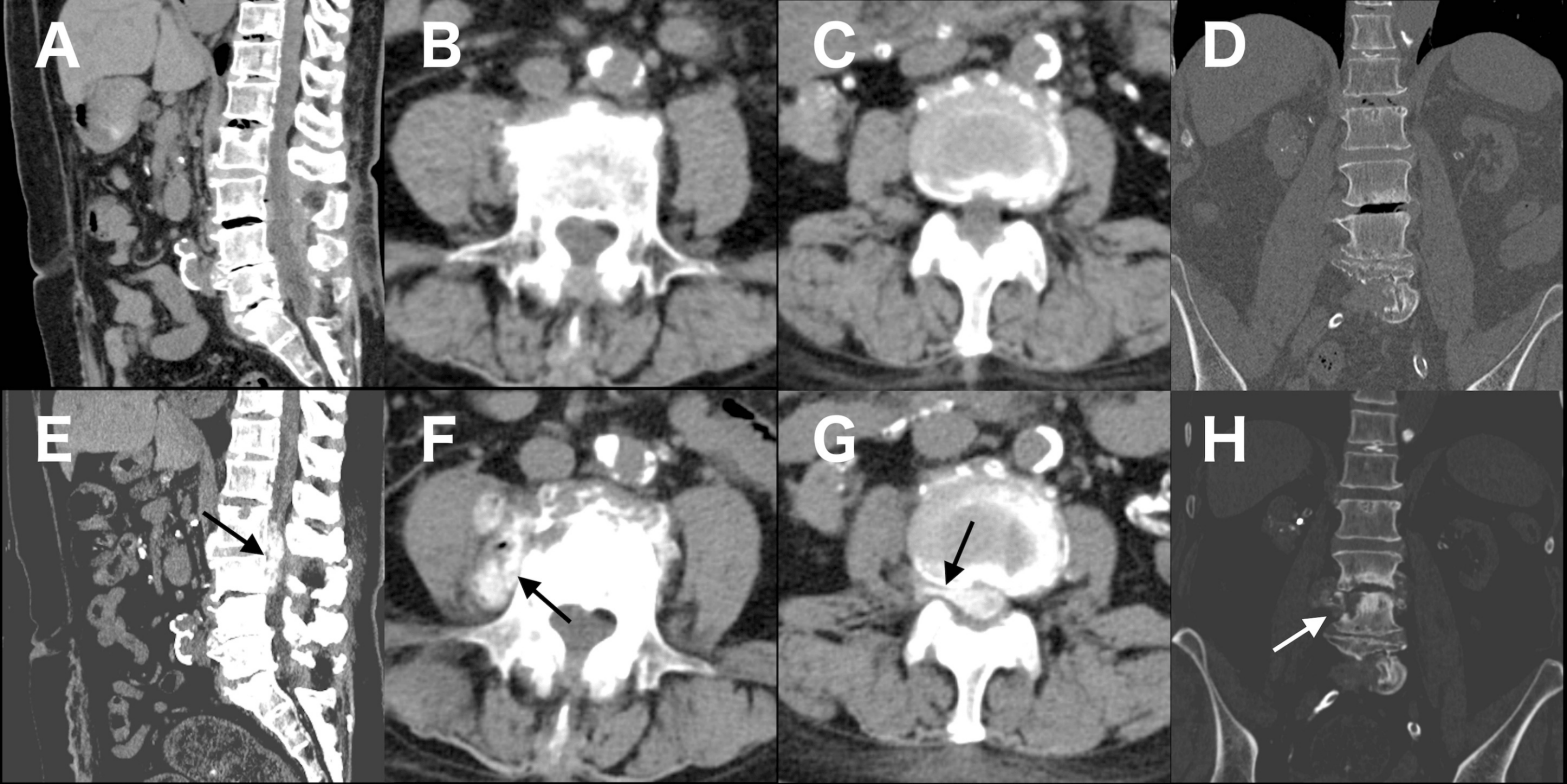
CT findings three months before admission (panels A-D) and on admission (day 0) (panels E-H). A, E: Sagittal images showing epidural calcification at L2–3 (arrow); B, F: Axial images showing perivertebral calcification (arrow); C, G: Axial images showing calcification in the right L2/3 intervertebral foramen (arrow); D, H: Axial images showing destructive changes in the L4 vertebral body (arrow) Panels A, E; B, F; C, G; and D, H represent the same anatomical levels for comparison, demonstrating the progression of calcification and vertebral destruction over time.
